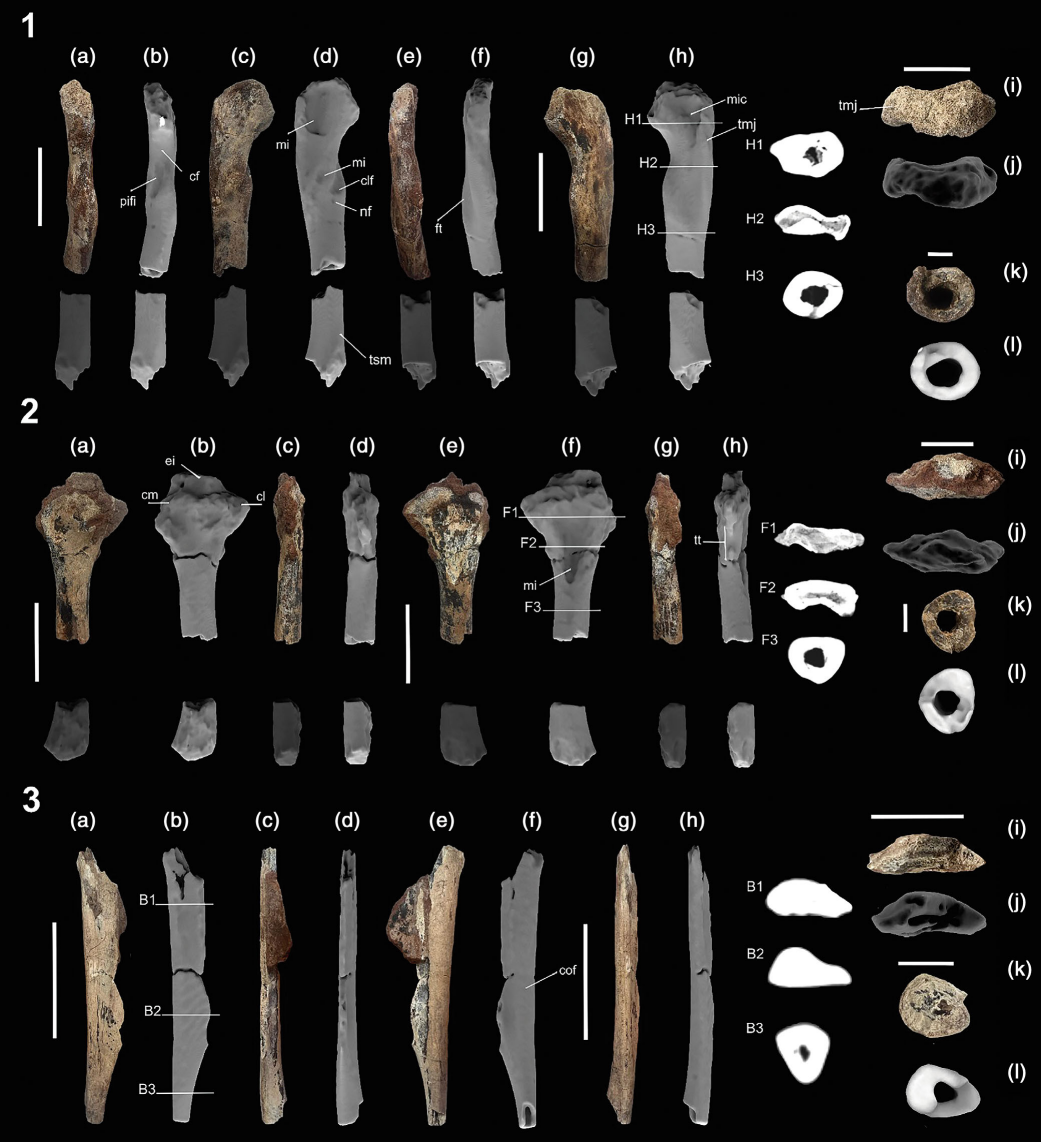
Pissarrachampsa* LPITB‐PV 57, photographs (a, c, e, g, i, k) and 3D models created from computed tomography (CT) scan (b, d, f, h, j, l). (1) *Left femur* in anterior (a, b), medial (c, d), posterior (e, f), lateral (g, h), proximal (i, j) and distal (k, l) views. (h1–h3), Cross‐sections of the CT scan (shown in 1h). (2) *Right tibia* in anterior (a, b), medial (c, d), posterior (e, f), lateral (g, h), proximal (i, j), and distal (k, l) views. (f1–f3) Cross‐sections of the CT scan (shown in 2f). (3) *Right fibula* in anterior (a, b), lateral (c, d), posterior (e, f), medial (g, h), proximal (i, j), and distal (k, l) views. (b1–b3) Cross‐sections of the CT scan (shown in 3b). cf, corpus femoris; cl, condylus lateralis; clf, *Musculus caudofemoralis longus*; cm, condylus medialis; cof, corpus fibulae; ei, eminentia intercondylaris; ft, fourth trochanter; mi, muscular insertion; mic, *M. ischiotrochantericus*; nf, nutrient foramen; pifi, *M. puboischiofemoralis internus*; tmj, trochanter major; tsm, tuberositas supracondylaris medialis; tt, tuberositas tibiae. Scale in (a–h) = 50 mm; (i and j) = 20 mm, (k and l) = 10 mm. h1–h3, f1–f3, b1–b3 = not to scale.
